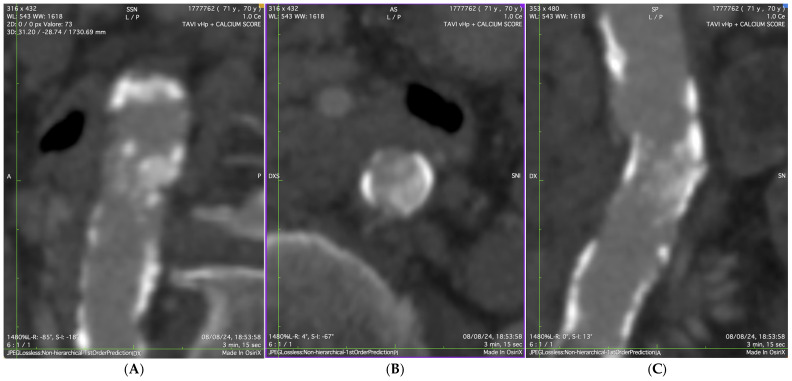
Multiplanar CT reconstructions of the subrenal abdominal aorta showing extensive calcification. (A) coronal view; (B) axial (transverse) section demonstrating concentric calcific stenosis; (C) sagittal view.
